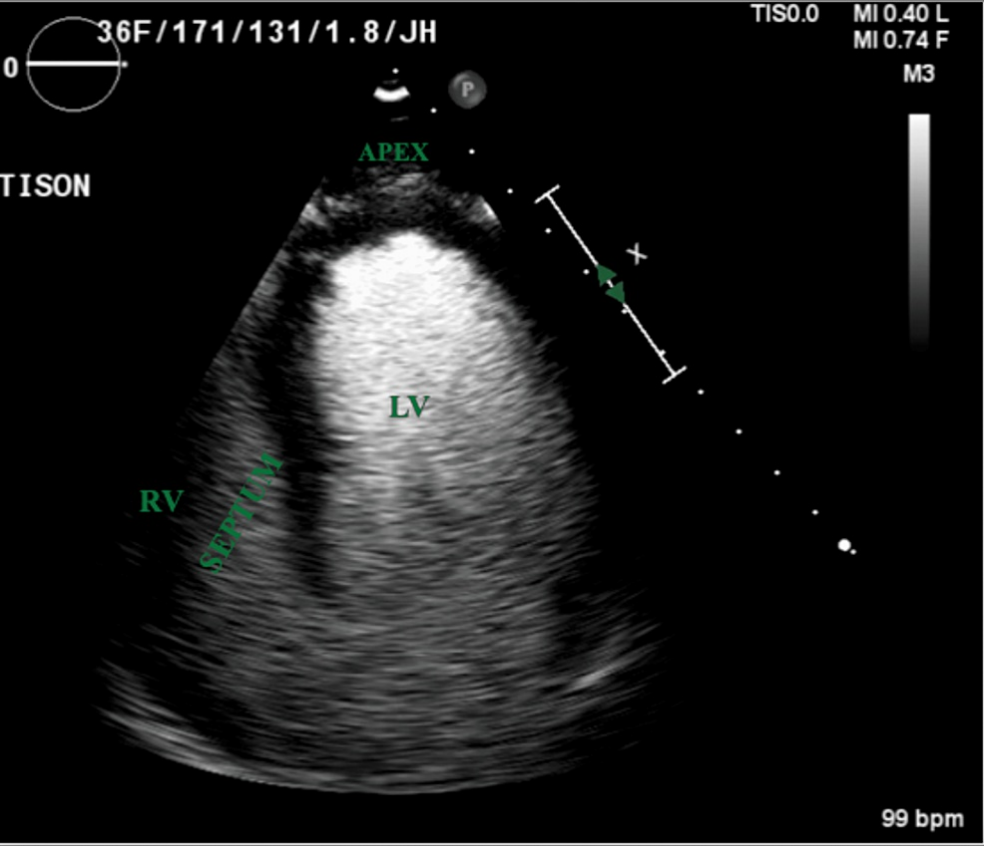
Echocardiogram showing apical ballooning in diastole LV: left ventricle, RV: right ventricle
